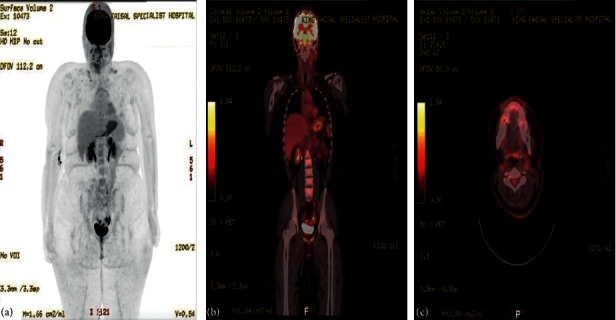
The PET scan in January 2022. The study ((a)–(c)) was compared with previous studies. The upper and lower cervical lymph nodes are showing mild FDG avidity with SUVmax of 1.8. However, there is no change morphologically, and it is most likely reactive. On the other hand, physiological radiotracer distribution is seen in the brain and there are no new suspicious FDG-avid lesions seen otherwise.
